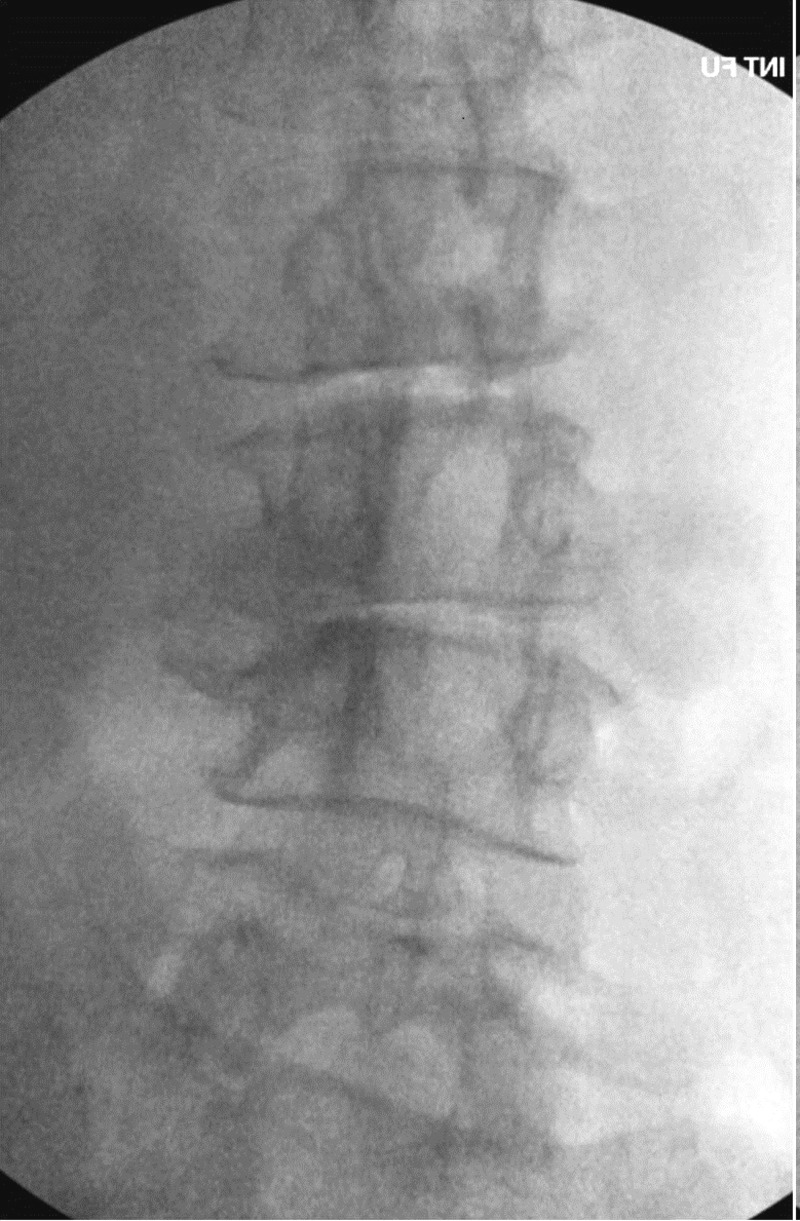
Preoperative x-ray Levoscoliosis L2-3, L3-4
